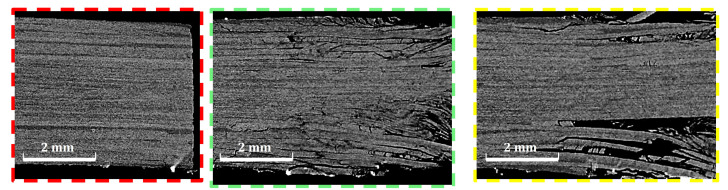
Computed Tomography scan of the first alternative (color code is in Figure 7).
